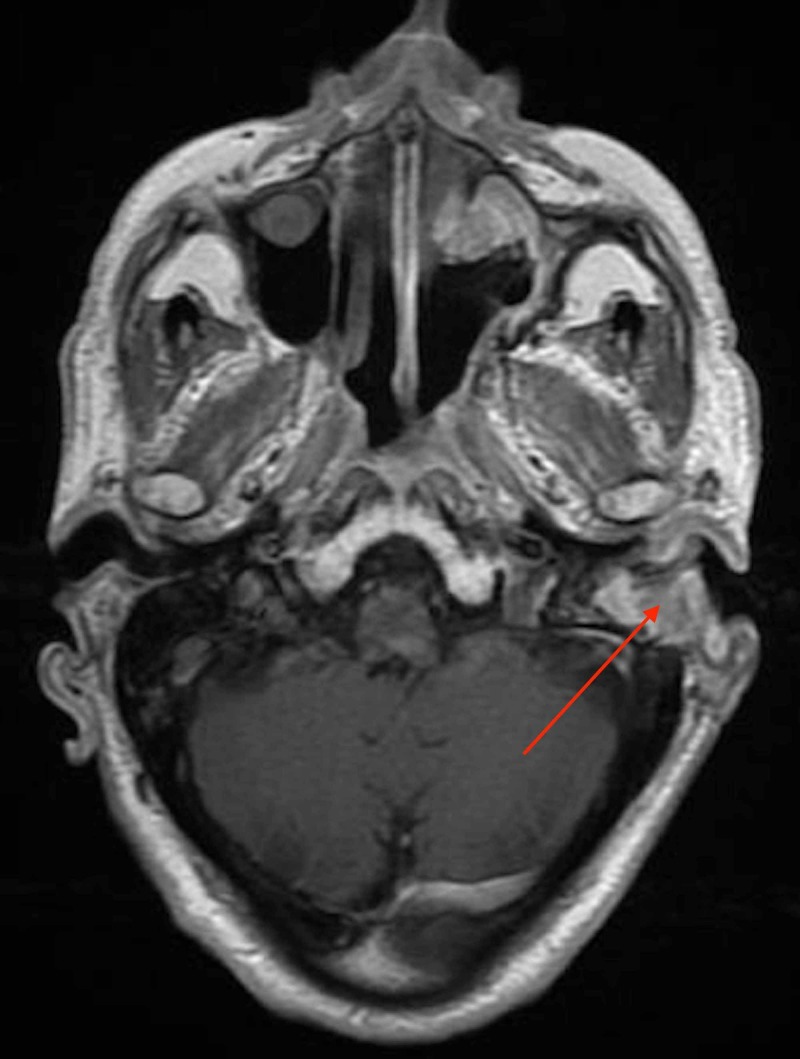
Post-operative axial MRI of the head showing interval progression of the external ear canal thickness.
